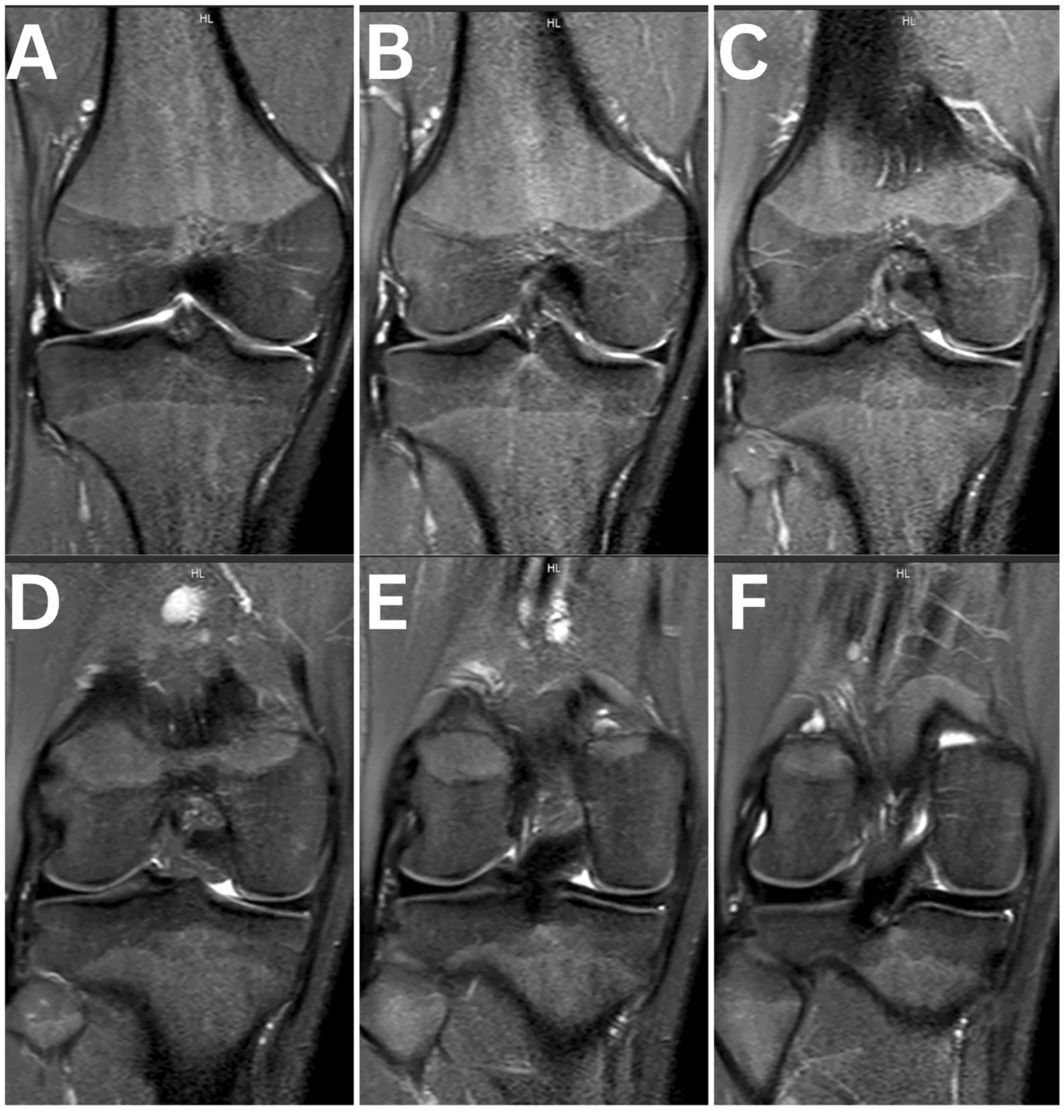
Follow‐up coronal MRI at 12 months. Coronal fat‐suppressed proton density–weighted images (A–F, anterior to posterior) demonstrate restoration of ACL continuity, with a continuous low‐signal band of normal oblique orientation extending from the femoral notch to the tibial intercondylar region. The prior empty‐notch appearance and heterogeneous ligament remnant are absent. Only trace joint fluid is present; the PCL, collateral ligaments, and menisci are unremarkable.
